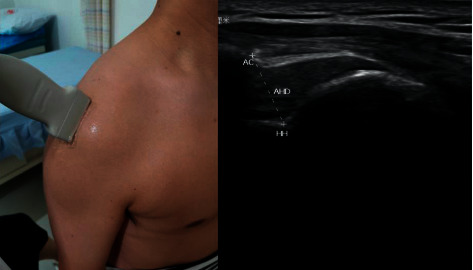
Measurement of acromiohumeral distance (AHD) for shoulder subluxation by using musculoskeletal ultrasound imaging technique. AC indicates acromion; HH indicates humerus head.
